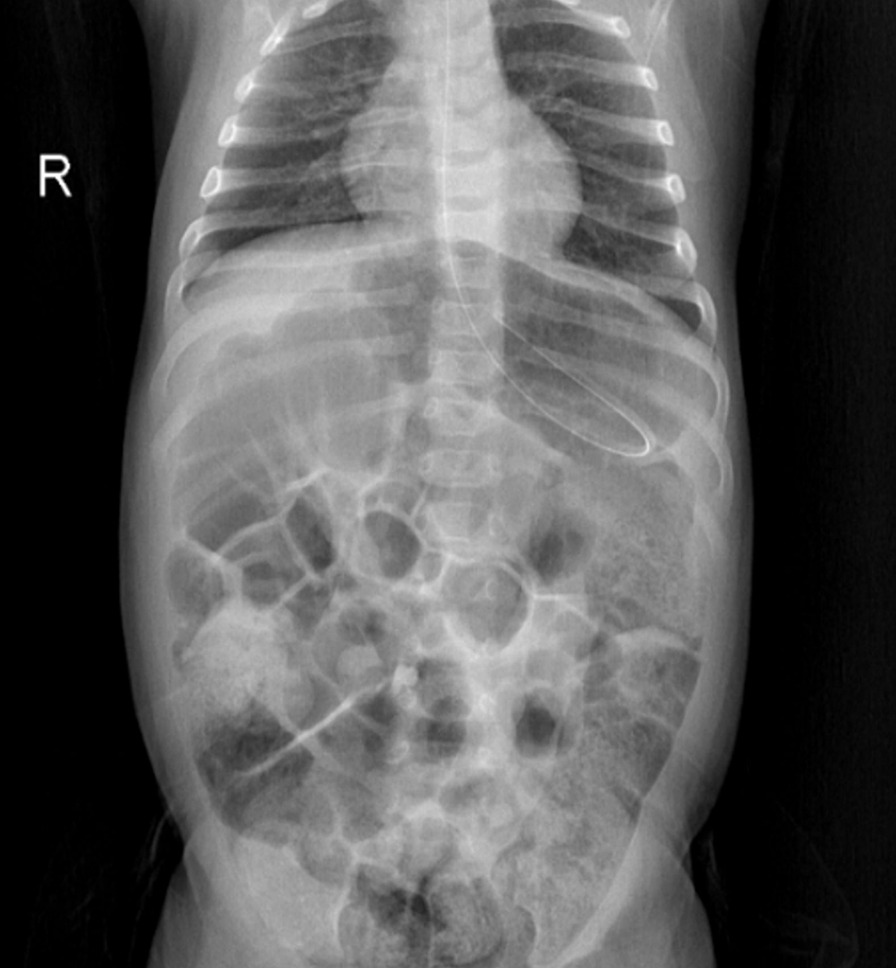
Excessive gas shadows in intestines and abnormally located fundus gas on the abdominal radiograph
